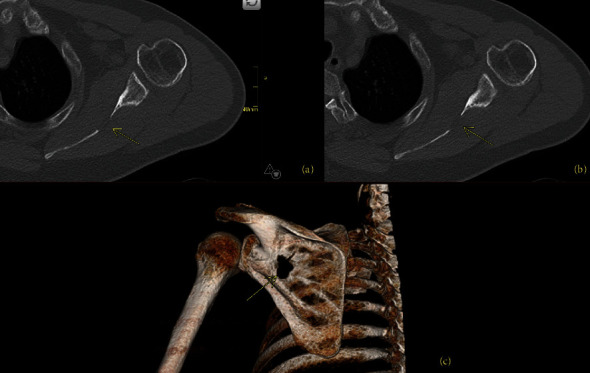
Case 1: left scapula CT and reconstruction images. Axial (a and b) and 3D reconstruction (c) CT images demonstrating the primary bone PMT arising from the left scapular body (arrow) in the patient from Case 1, corresponding to the findings from the DOTATATE-PET/CT in Figure 1.
